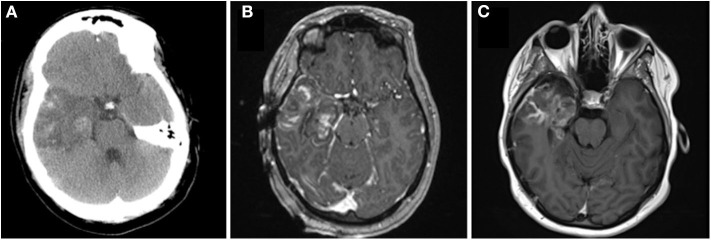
Panel demonstrates intra-operative and peri-operative patient imaging. (A) Axial CT obtained on post-operative day 2 demonstrates cerebral edema at the ablation site. (B) Post-ablation contrast-enhanced T1 axial MRI that demonstrates ablation of temporal lobe from the temporal pole to the level of the lateral mesencephalic sulcus and collicular plate at the time of surgery. (C) Contrast-enhanced (fluid dark) T1 axial MRI demonstrates the ablation 3 months after surgery.
